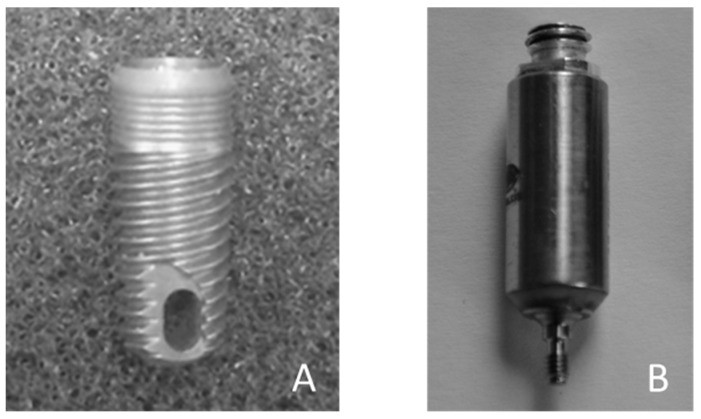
(A) Dental implant (Zimmer Biomet, TSVT4B10); (B) Planar ultrasonic contact transducers generating a 10 MHz broadband ultrasonic pulse.
